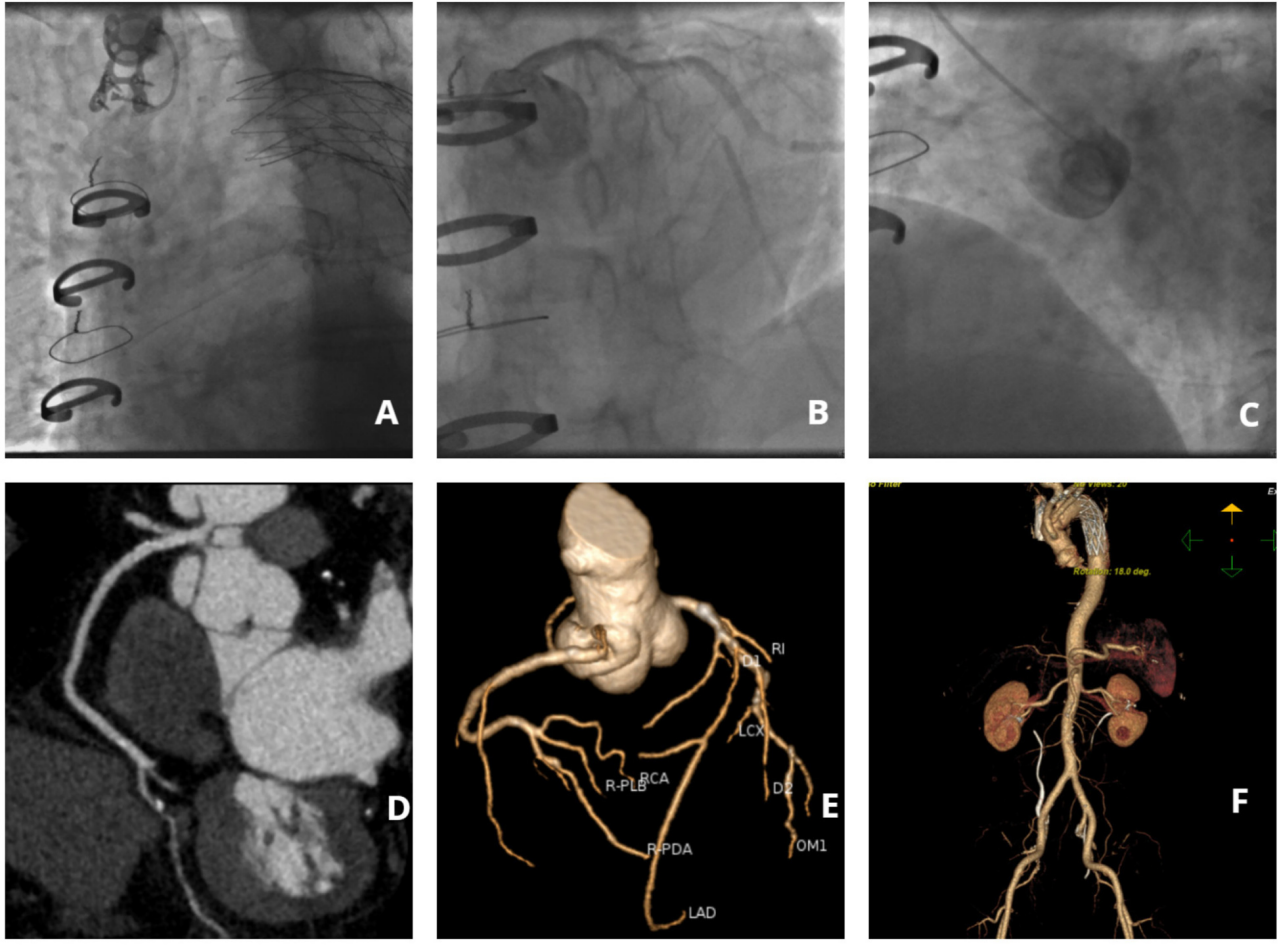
Imaging findings of coronary and aortic vasculature. (A) Angiographic visualization of brachiocephalic trunk tortuosity; (B) Selective angiography of the left coronary artery; (C) Non-selective right coronary angiography performed via pigtail catheter; (D) Computed tomography angiography (CTA) of the right coronary artery; (E) Coronary CTA; (F) Aortic CTA.
